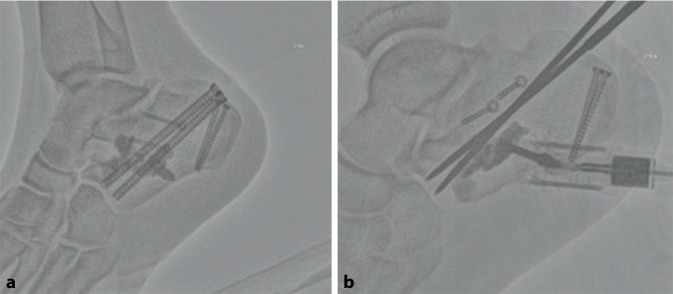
Auch bei komplexeren und/oder kombinierten Frakturmorphologien kann diese Technik angewandt werden. Hierbei empfiehlt es sich, z. B. zunächst die Tongue-Type-Komponente der Pars posterior ebenfalls perkutan über kleine Stichinzisionen zu reponieren und präliminar mit Bohrdrähten oder einer Repositionszange zu halten. Es schließt sich das Einjustieren des ggf. vorher wiederhergestellten Gelenkblocks gegen die korrespondierende Gelenkfläche des Talus mittels Ballonkatheter an. Die gesamte Versorgung erfolgt minimalinvasiv über kleine Stichinzisionen von lateral. Eventuell aus dem Defekt ausgetretener Zement sollte umgehend entfernt werden, um mechanischen Irritationen hierdurch vorzubeugen. Grundsätzlich könnte der eingebrachte Zement den Frakturlinien folgend nach medial, plantar, lateral und in das Subtalargelenk austreten; dies ist in der Fallserie nicht vorgekommen. Ein entsprechend vorsichtiges Einbringen des Zements unter Viskositätskontrolle via Radiofrequenztechnik und Durchleuchtung kann unerwünschte Extravasate verhindern. Bei der abschließenden Bildgebung des operativen Ergebnisses ist auf die korrekte Einstellung des Gissane-Winkels in der seitlichen Durchleuchtung sowie eine stufenfreie Abbildung in der Broden-Aufnahme zu achten (Abb. 5). Abb. 6 zeigt beispielhaft einen abschließenden Scan mit dem Durchleuchtungsroboter (Abb. 6). Die Weiterbehandlung erfolgt für 8 Wochen in einer rückfußentlastenden Orthese (z. B. nach Settner) unter medikamentöser Thromboembolieprophylaxe mit regelmäßigen Blutbildkontrollen (z. A. HIT-II-Reaktion). Vier und 8 Wochen postoperativ werden konventionelle Röntgenkontrollen angefertigt (seitlich, axial und Broden-Projektion), im Bedarfsfall eine CT.
